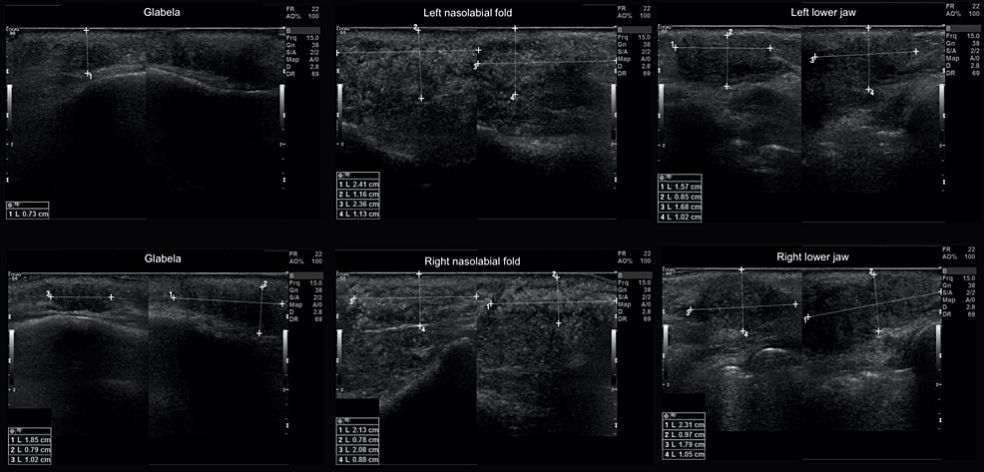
Ultrasound images identifying the dimensions and location of the dense, clinically palpable material.
